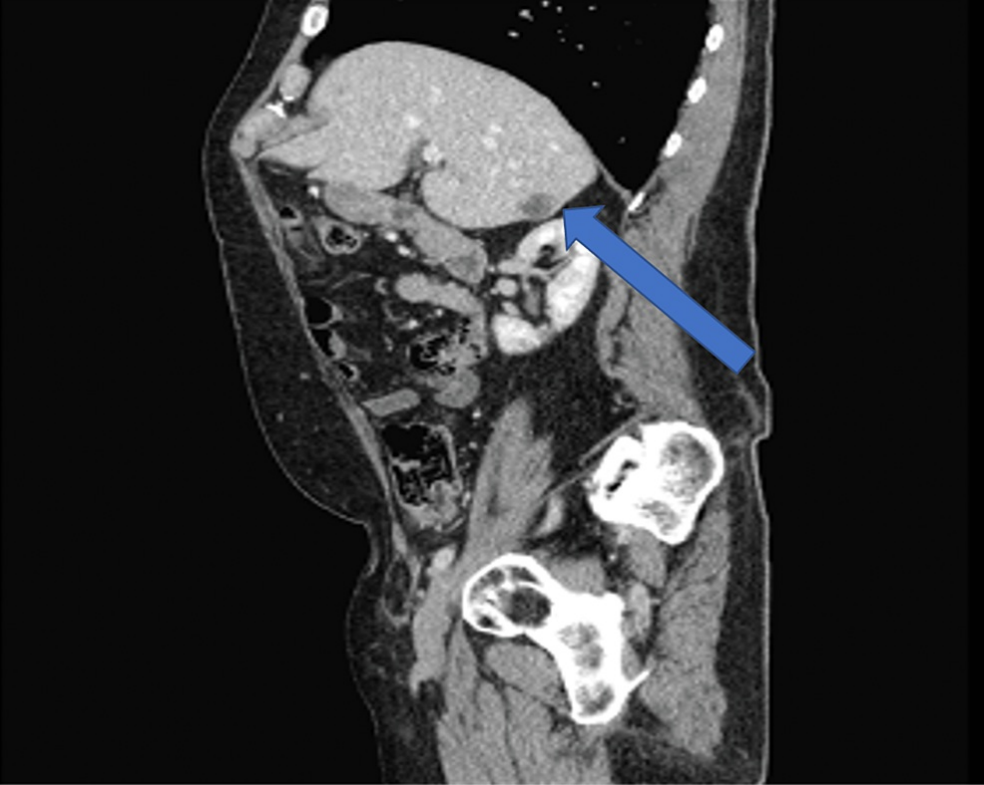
CT abdomen and pelvis triple-sagittal view phase demonstrating a 2.6-centimeter lesion in liver segment 6
